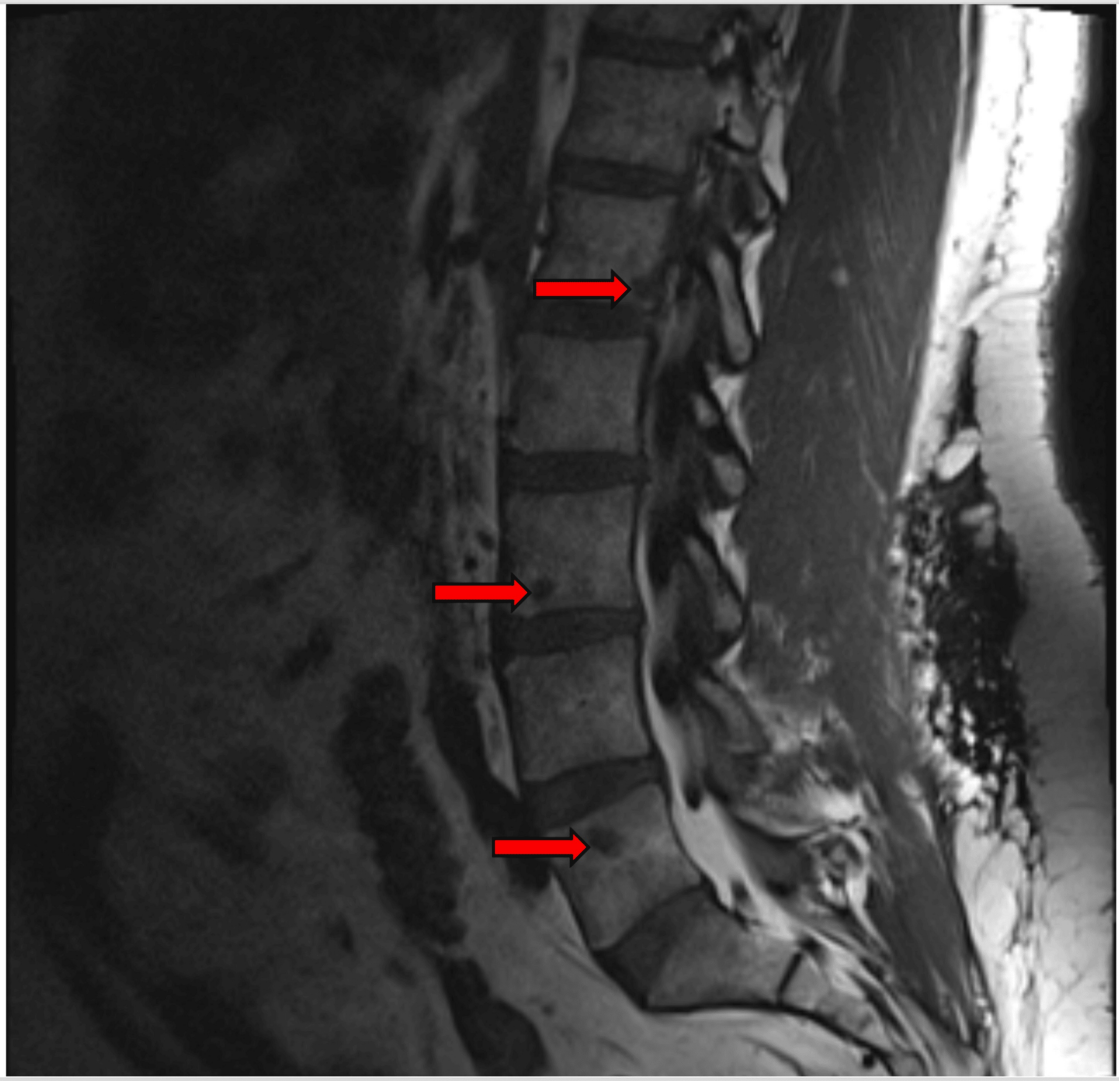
MRI of the lumbar spine showing multifocal hypointense lesions consistent with osseous sarcoidosis Three lesions are indicated by red arrows.
